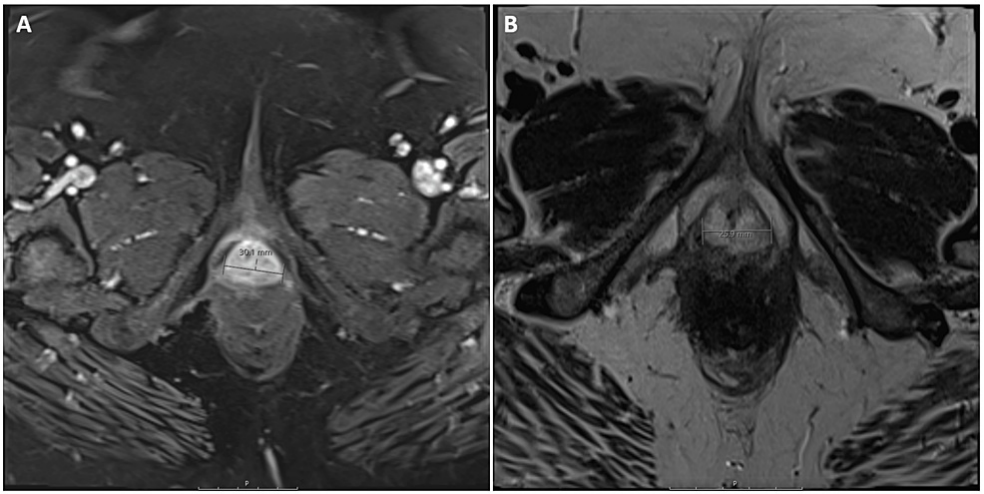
MRI findings MRI of the pelvis, T1-weighted (A) and T2-weighted (B), demonstrated a residual mass at the urethral meatus, performed approximately three weeks after initial resection.
